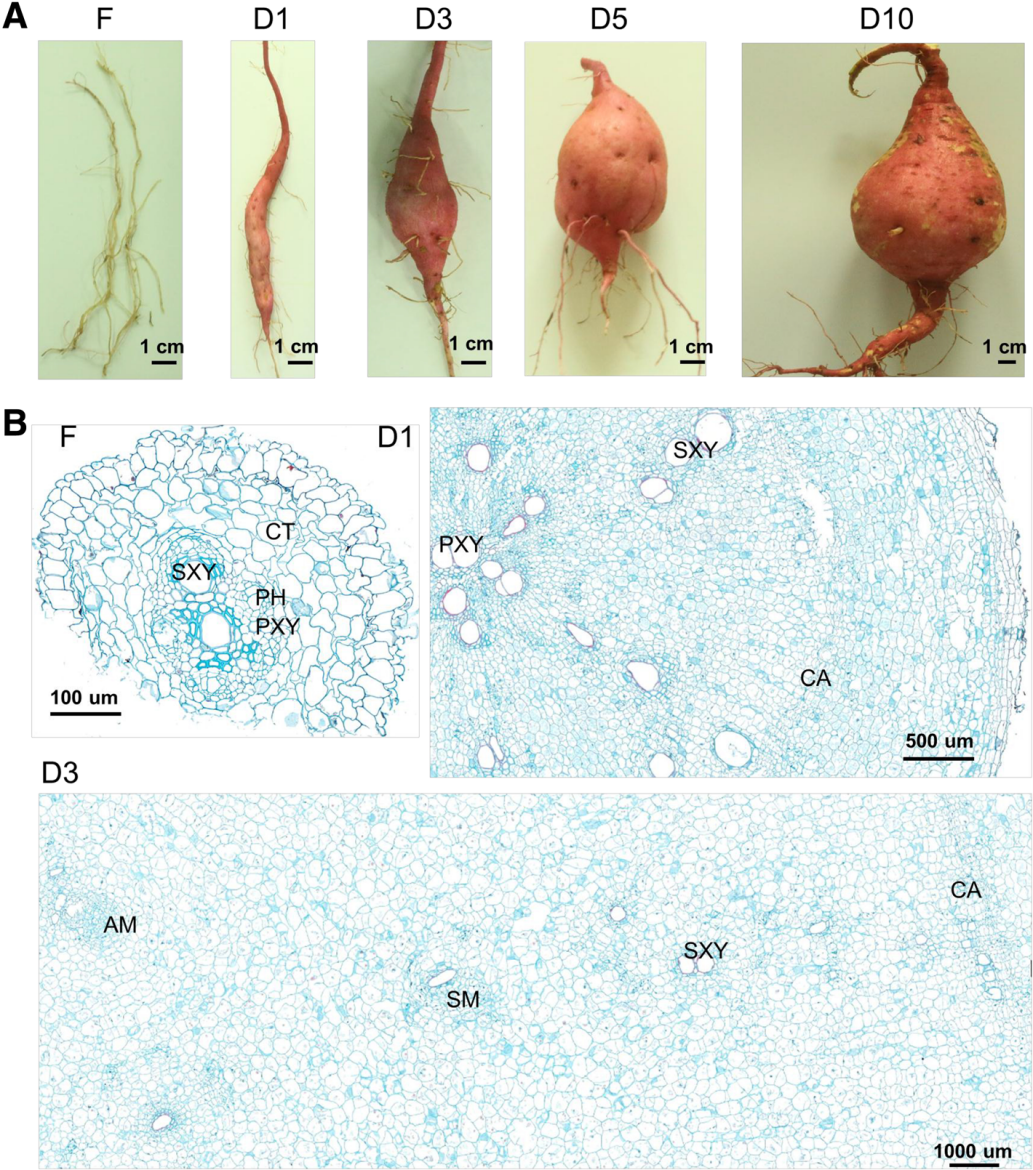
Morphology and anatomy of fibrous roots and sweet potato storage roots at different stages. a Phenotypic characterization of fibrous roots and storage roots at different stages. b Transverse sections of fibrous roots and storage roots at different stages. F, fibrous root (diameter of approximately 1 mm); D1, initial storage root (diameter of approximately 1 cm); D3, storage root (diameter of approximately 3 cm); D5, storage root (diameter of approximately 5 cm); D10, storage root (diameter of approximately 10 cm); CT, cortex; PH, phloem; PXY, protoxylem; SXY, secondary xylem; CA, cambium; SM, secondary meristem; AM, anomalous meristem
